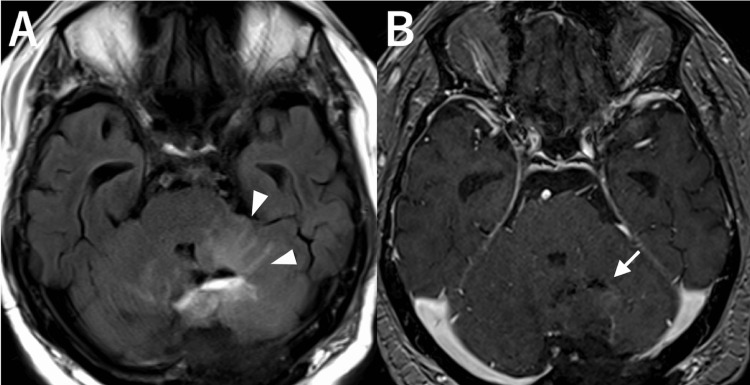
Postoperative images. A, B: Postoperative magnetic resonance imaging demonstrating significant resolution of perilesional edema on fluid-attenuated inversion recovery imaging (A, arrowheads) and complete removal of the contrast-enhanced lesion on T1-weighted imaging (B, arrow).
